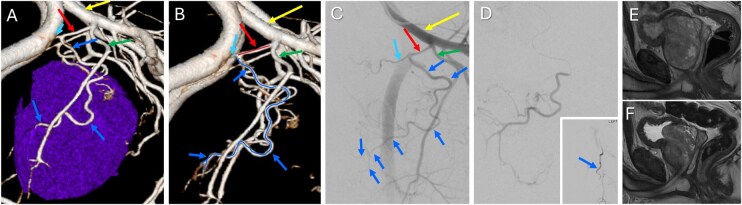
A 59-year-old male underwent preprocedural PCD-CTA for prostatic artery embolization. (A, B) High-resolution PCD-CTA 3D reconstructions (left pelvic oblique views) demonstrating the pelvic vasculature with the prostate gland segmented in purple (A). The left internal iliac artery (yellow arrows), the anterior division of the internal iliac artery (green arrows), and the vesicoprostatic trunk (red arrows; overlay in B) arise from the anterior division. Distal branching shows the left prostatic artery (blue arrows; overlay in B) and the superior vesical artery (light blue arrows; overlay in B). (C, D) Corresponding digital subtraction angiography (DSA) images obtained during the embolization procedure confirm the same arterial anatomy; the inset in D shows successful catheterization and embolization of the prostatic artery. (E, F) Sagittal T2-weighted MRI images before and after embolization, respectively, showing interval reduction in prostate volume from 155 mL (E) to 90 mL (F) following successful PAE. High spatial resolution of PCD-CTA enabled confident delineation of complex pelvic arterial anatomy that was overlaid on the DSA images during the procedure using embolization guidance software. Abbreviations: Abbreviations: CTA, CT angiography; DSA, digital subtraction angiography; EID, energy-integrating detector; PAE, prostatic artery embolization.
